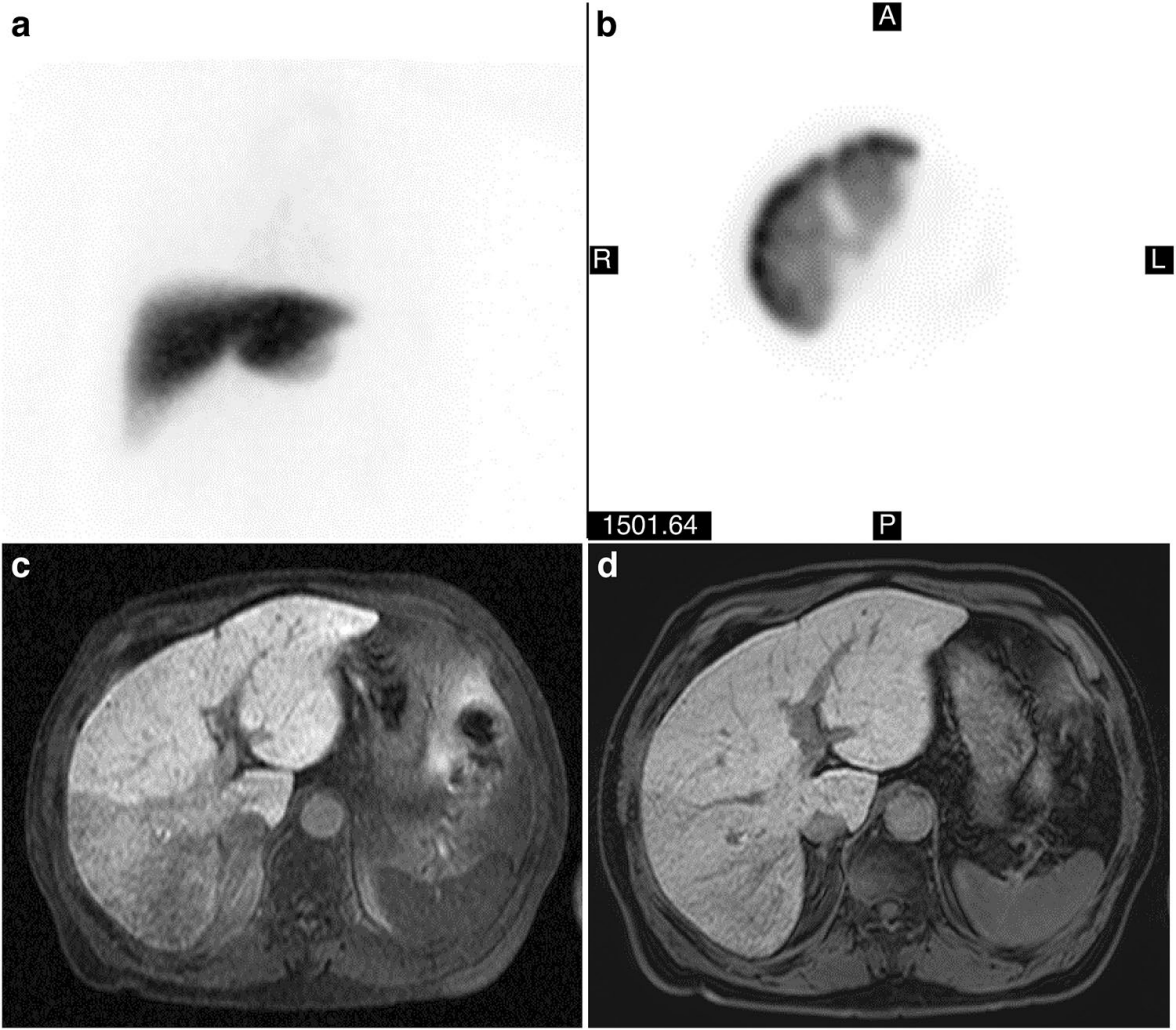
Comparison of ^99m^Tc-GSA liver scintigraphy and Gd-EOB-DTPA-enhanced MRI. (a) Planar image of ^99m^Tc-GSA liver scintigraphy. (b) Transverse image of ^99m^Tc-GSA liver scintigraphy. It is impossible to distinguish hepatic segments from this image because intrahepatic portal vein and hepatic vein were not imaged. (c) Gd-EOB-DTPA-enhanced MRI with a built-in body coil. A decrease in signal intensities in right posterior segments was clearly imaged, which is not found in (a,b) images. (d) Gd-EOB-DTPA-enhanced MRI with a 32ch phased array coil. The contrasts between right anterior and posterior segments decreased compared with the (c) image.
